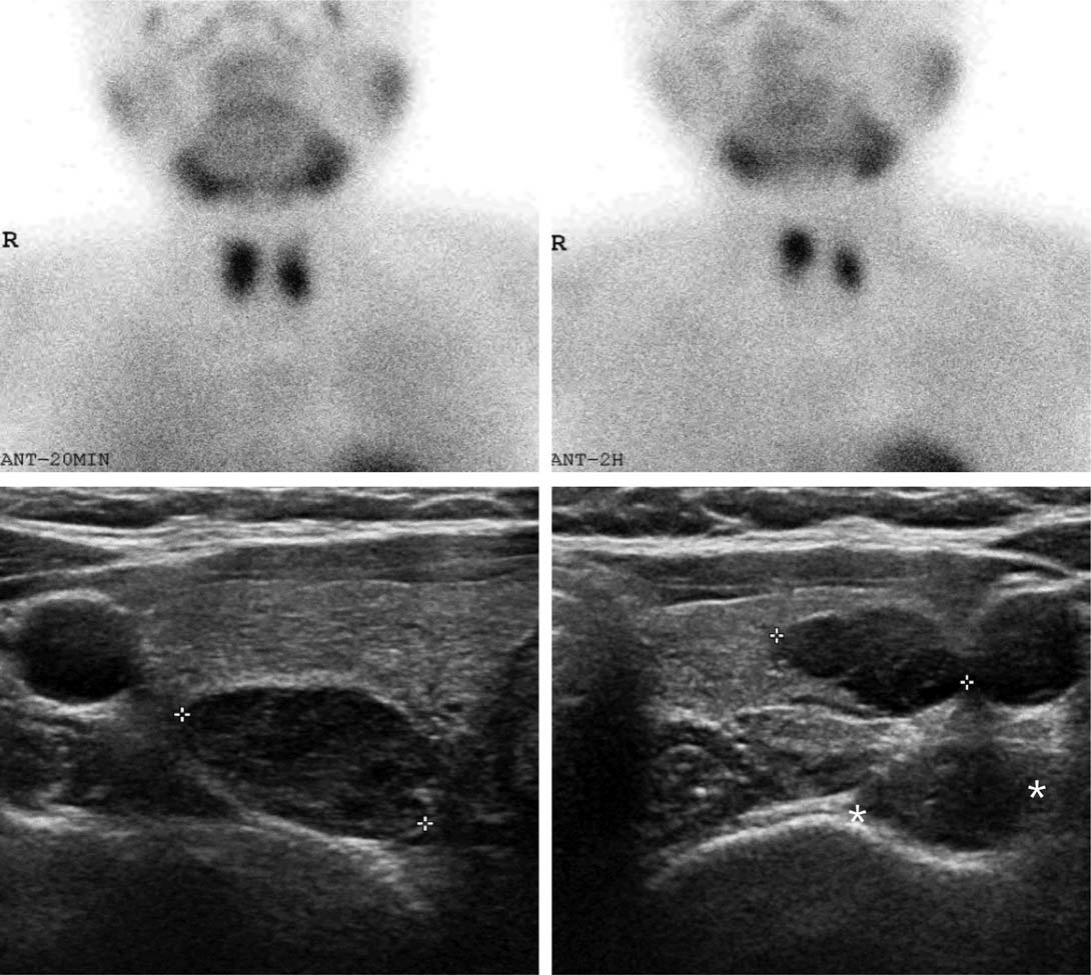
Right and left inferior parathyroid adenomas detected on ^99^Tc-sestamibi parathyroid scintigraphy (upper) and thyroid ultrasonography (lower).
